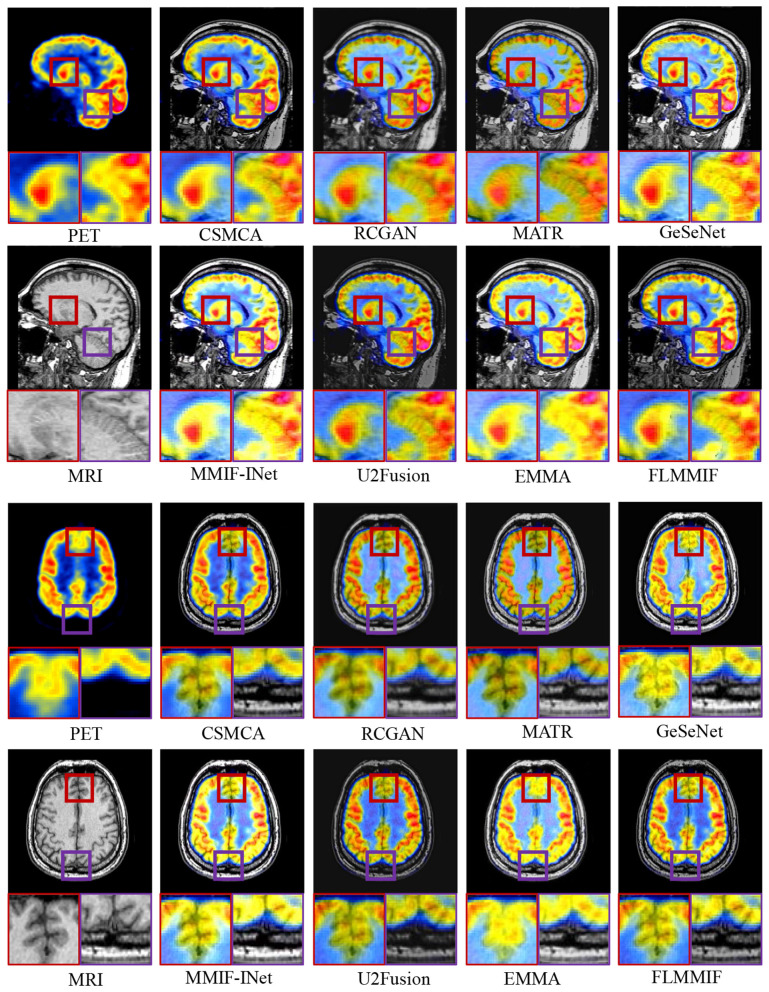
Qualitative assessment on PET-MRI dataset.
